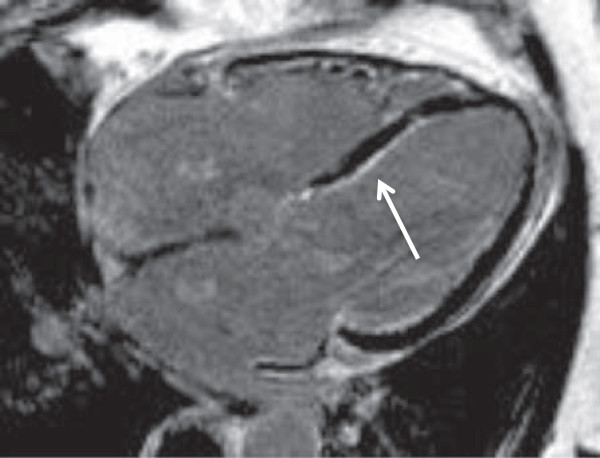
Magnetic resonance imaging with arrow depicting subendocardial delayed enhancement in the 4 chamber axial view.
